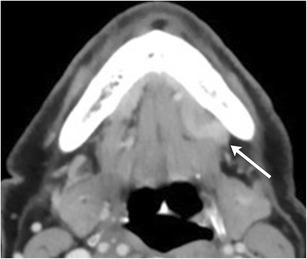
Sublingual gland herniation, mimicking a palpable “lesion” on physical exam. Axial contrast-enhanced CT image demonstrates herniation of the left submandibular gland through a mylohyoid muscle sling defect
